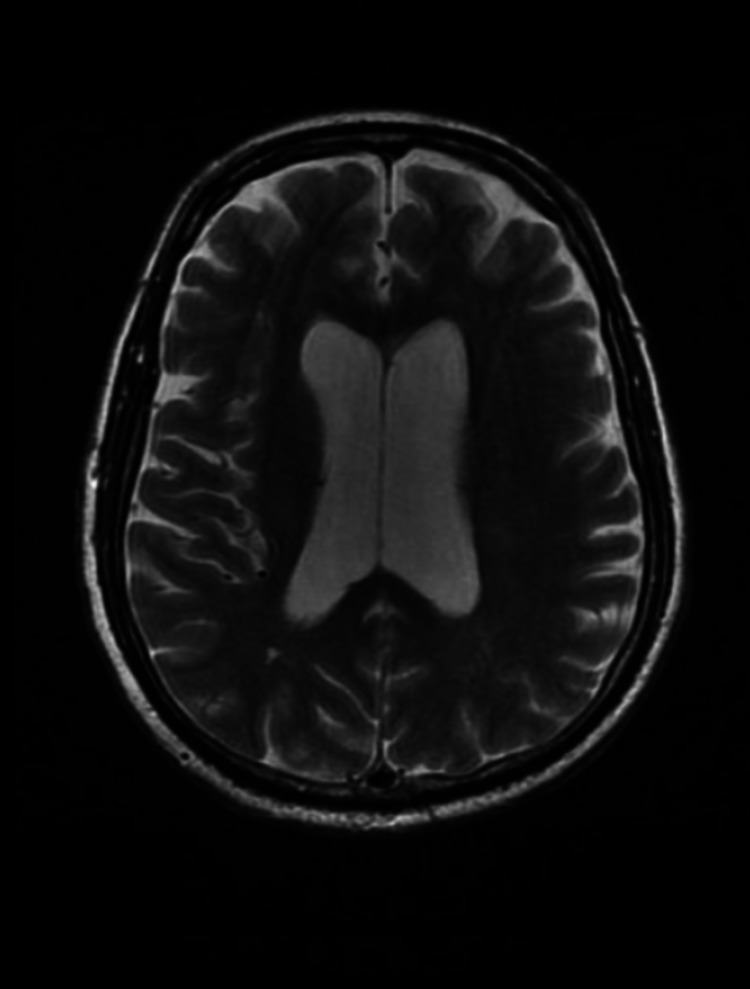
Axial T2 magnetic resonance imaging (MRI) showing ventricular mild ectasia.
